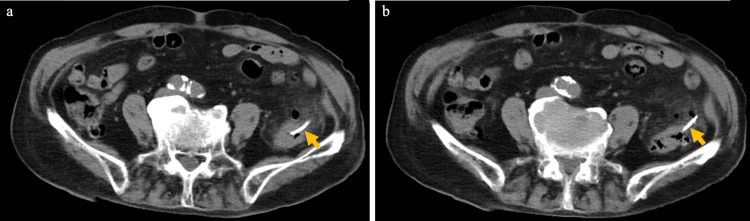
A perforation of the descending colon caused by the migrated PSDS On the 19th day, CT revealed perforation of the descending colon caused by the migrated PSDS (yellow arrows). CT, computed tomography; PSDS, pancreatic spontaneous dislodgement stent
